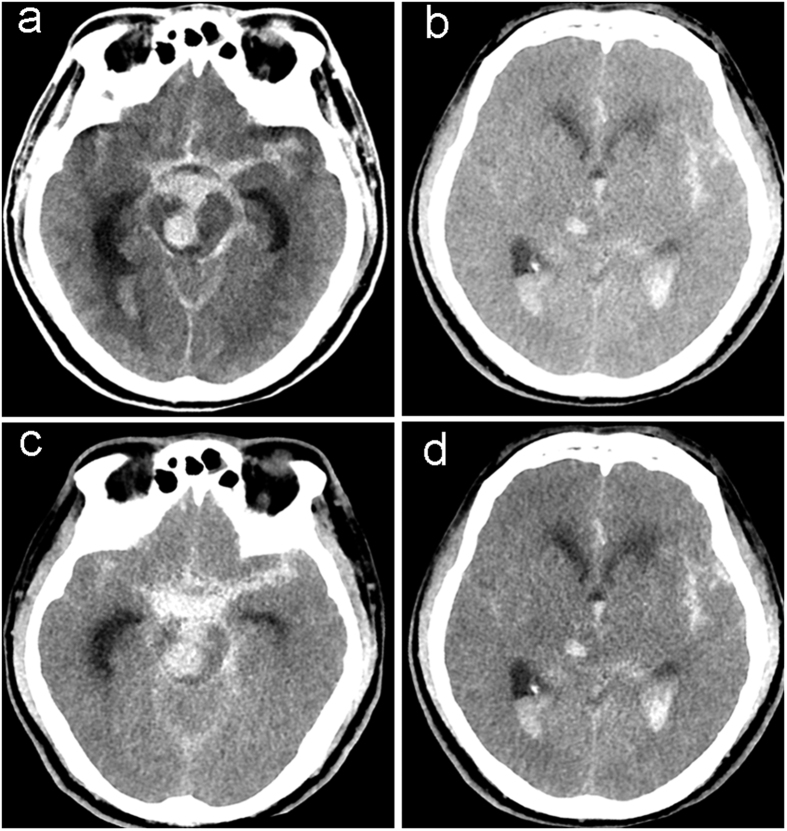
A 59-year-old man with spontaneous subarachnoid hemorrhage and ventricular hematoma caused by ruptured aneurysm of right anterior superior cerebellar artery. (a,b) True non-enhanced CT image, (c,d) virtual non-enhanced CT image.
